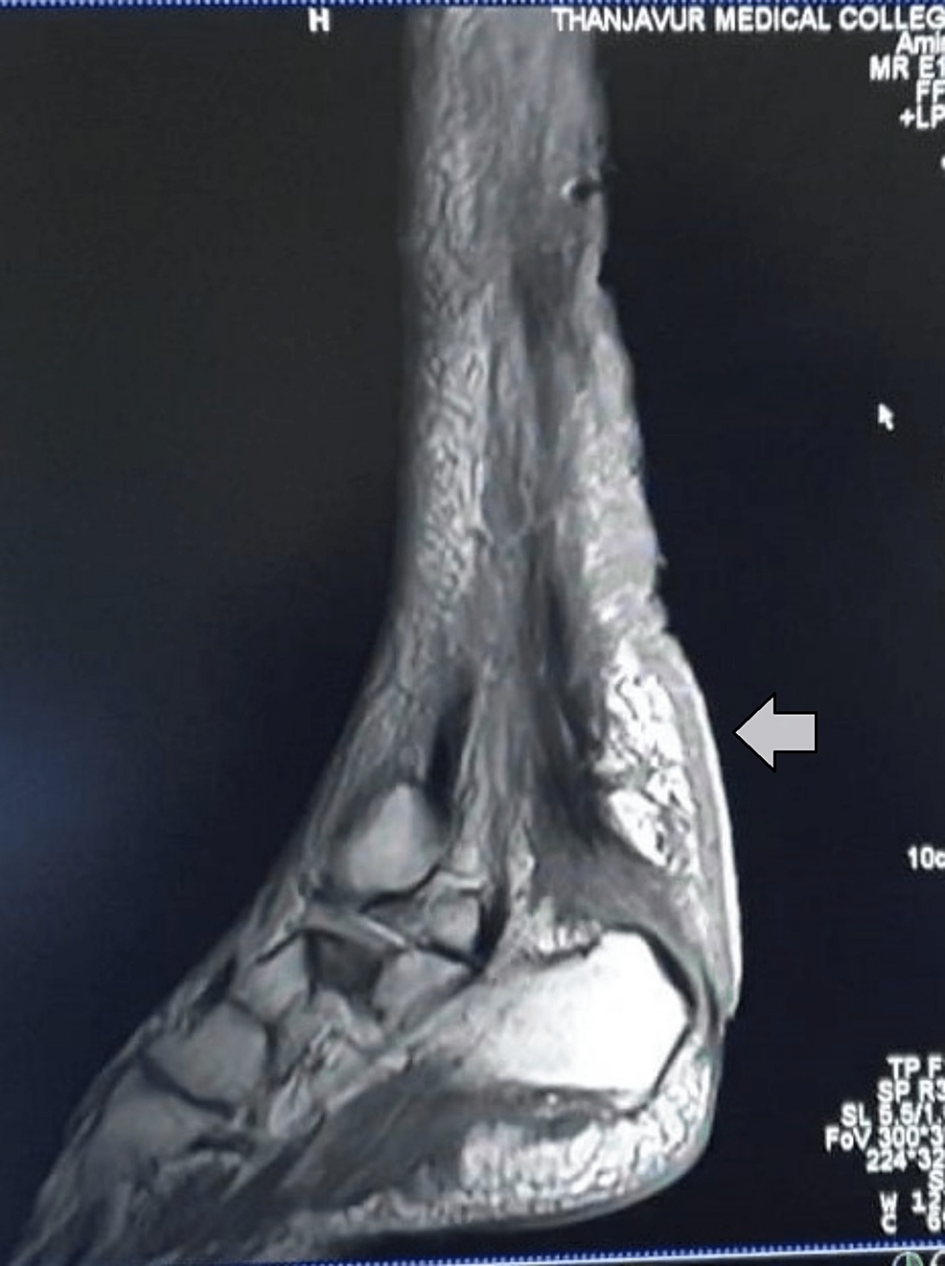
MRI of the right ankle in case 1 MRI T1 image in sagittal section showed tendon xanthoma with intermediate signal intensity in the tendo achilles (arrow)
